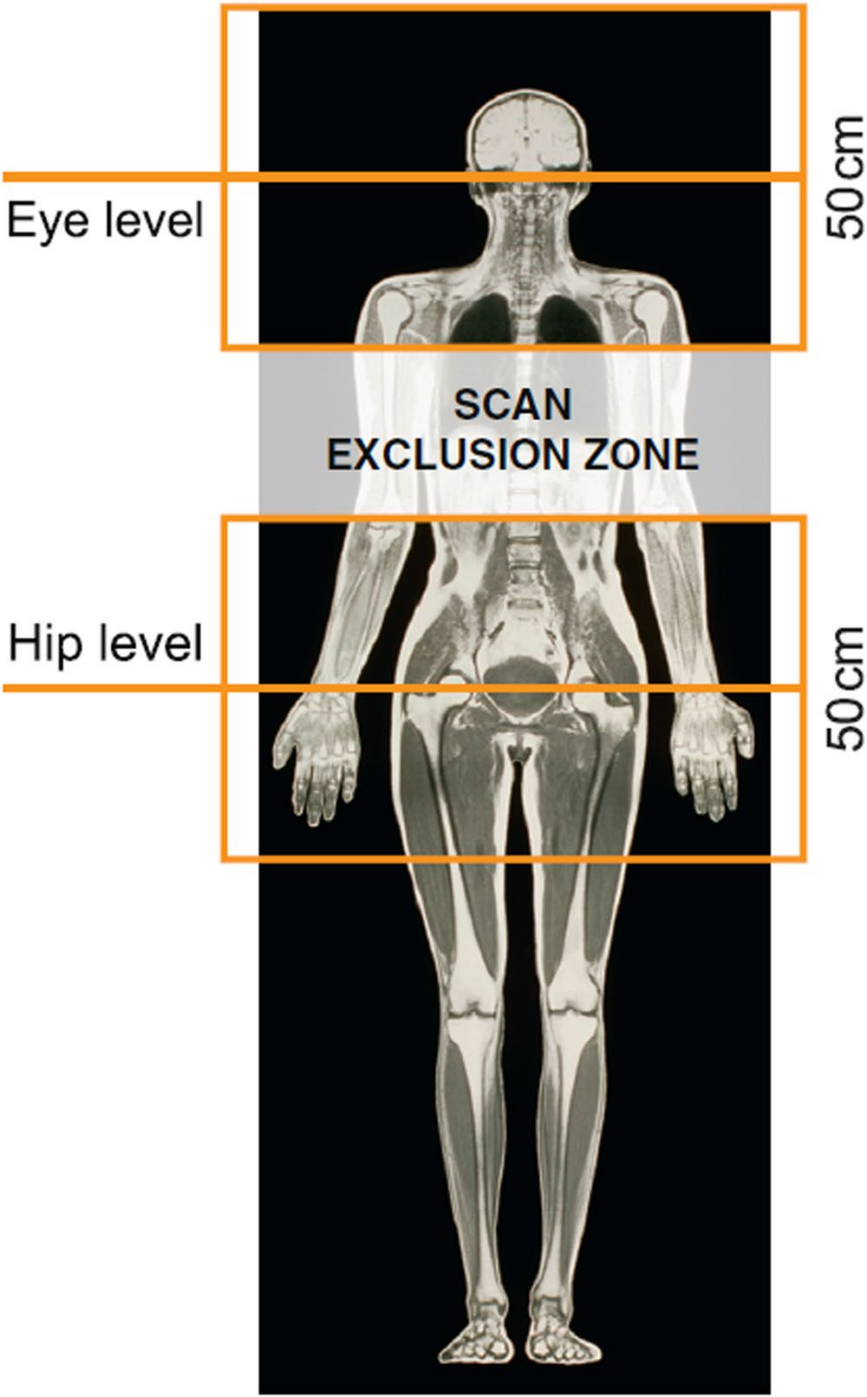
Isocentres at eye and hip level of magnetic resonance imaging scans, the available area for the scans (≈50 cm × 50 cm), and the scan exclusion zone. The size of the usually quadratic field of view varied with body region, scanner manufacturer (Siemens, Philips, GE), and the type of scan sequence (Table 2). The most common field of view was 23 cm × 23 cm, resulting in greater magnetic field gradients and thus more compelling testing conditions than it would be for a larger (e.g. 50 cm × 50 cm) field of view.
